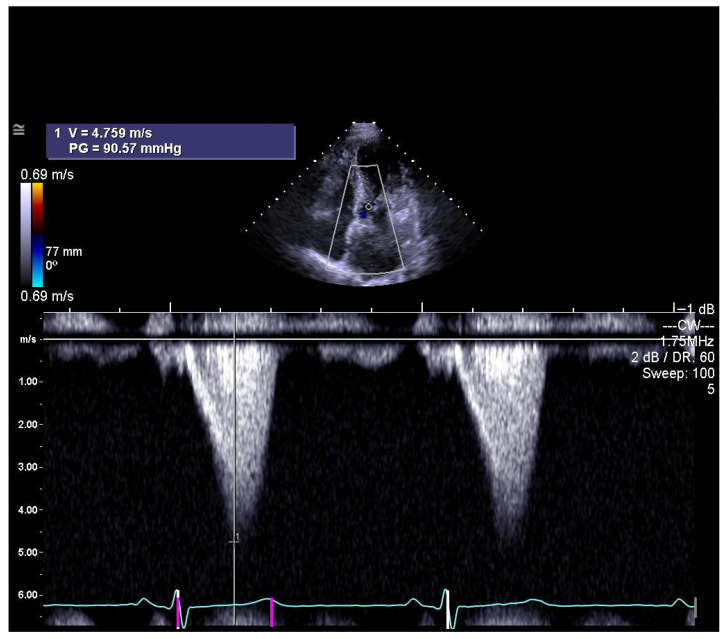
The left ventricular outflow tract (LVOT) shows a maximum gradient, usually with a late systolic peak, as assessed by continuous-wave Doppler echocardiography (photo and copyright: T.P.). PG—peak aortic valve gradient; V—maximum aortic valve velocity.
